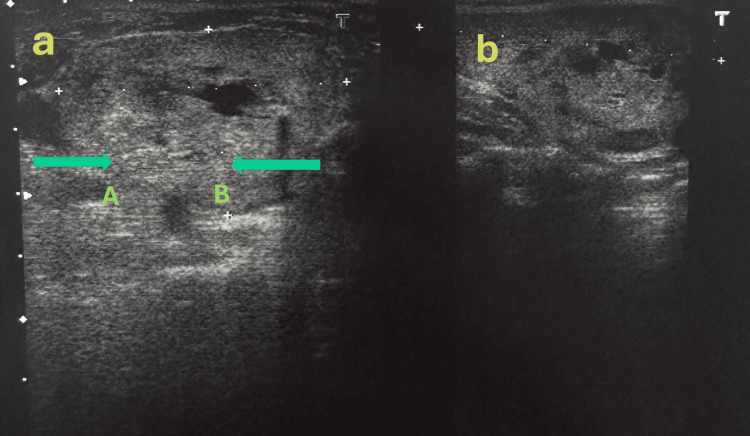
Ultrasonography showing parathyroid adenoma in the lower left region (green arrows delineate the respective zones evaluated in both axes) (a) Transverse ultrasound image showing two adjacent regions of interest: (A) a hypoechoic area suggestive of parathyroid adenoma and (B) a comparatively isoechoic or hyperechoic area, possibly representing surrounding tissue and normal parenchyma; (b) Corresponding longitudinal view of the same anatomical structures.
